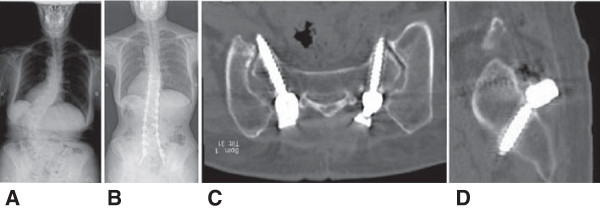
Radiographs of a 72-year-old female with degenerative scoliosis. A: Posteroanterior view of the preoperative whole spine. B: Posteroanterior view of the postoperative whole spine. C: Reconstructed image of the plane along the axes of the intrasacral screws. D: Reconstructed sagittal image of the plane along the axis of the intrasacral screw.
